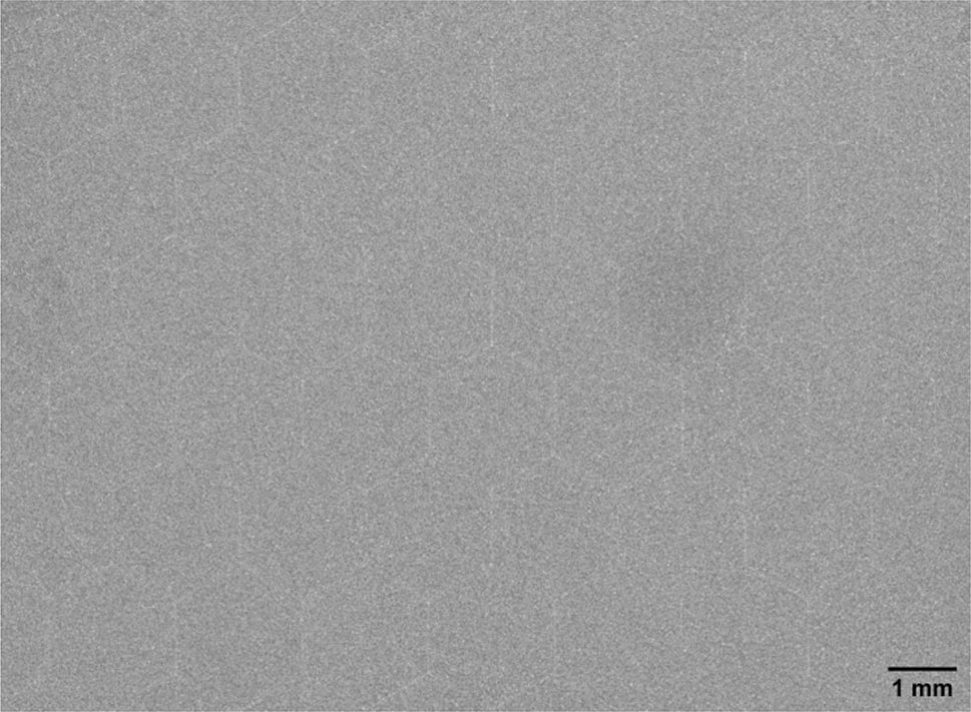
Background X-ray image of the PEALPB camera.
